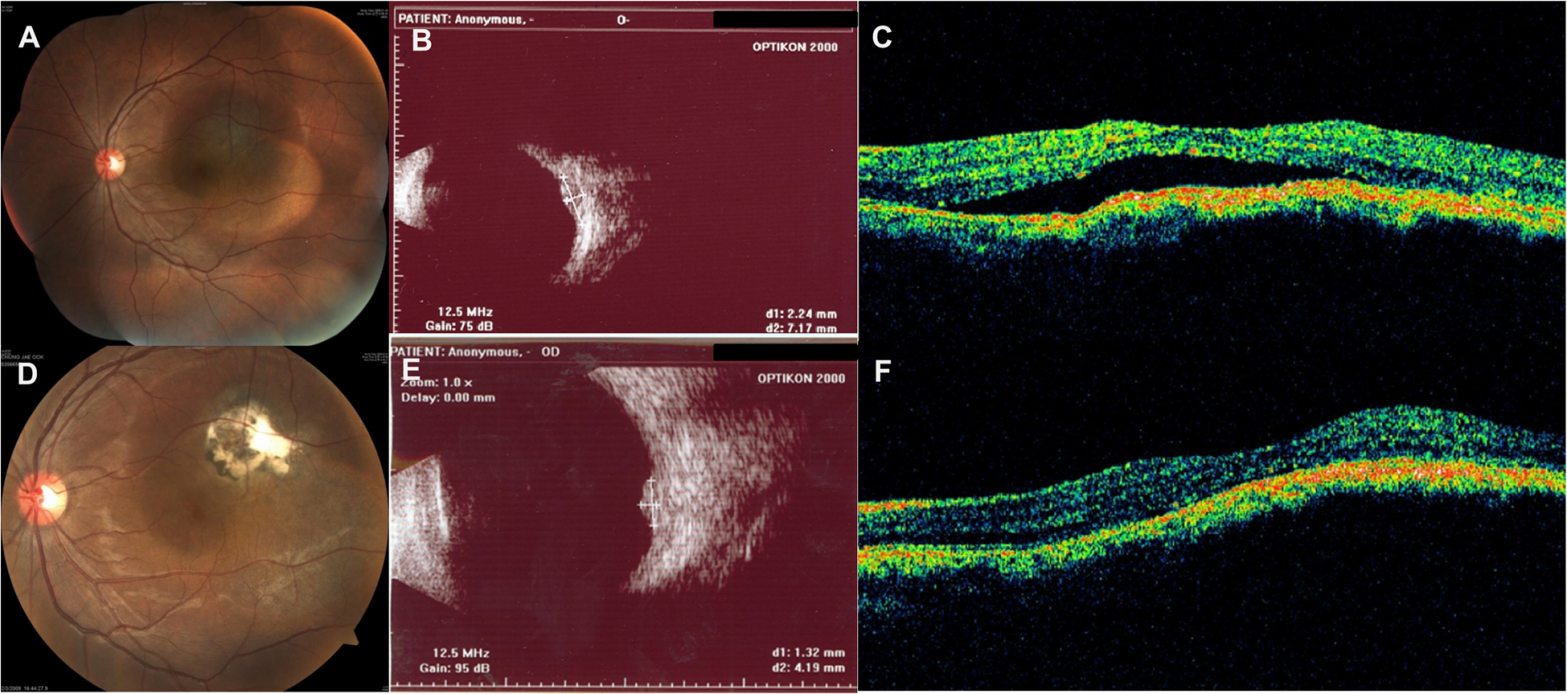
Treatment results of transpupillary thermotherapy for subfoveal fluid associated with choroidal melanocytic lesions. A 23-year-old man (Case 15) presented with 2-year history of decreased visual acuity of the left eye. His initial best corrected visual acuity (BCVA) was 20/63. a There was a pigmented subretinal mass supero-temporal to the fovea, b with a height of 2.24 mm and base diameter of 7.17 mm, as measured by B-scan ultrasonography. c Initial optical coherence tomography showed subretinal fluid at the macula. f After four sessions of transpupillary thermotherapy, subretinal fluid completely resolved and BCVA improved to 20/25. d The tumor showed scarring changes, and (e) tumor size decreased to 1.32 mm (height) by 4.13 mm (base diameter), as measured by B-scan ultrasonography. Improved vision and attached retinal status were maintained for 46 months after the last treatment. Tumor size further decreased to 0.50 mm (height) by 4.18 mm (base diameter)
